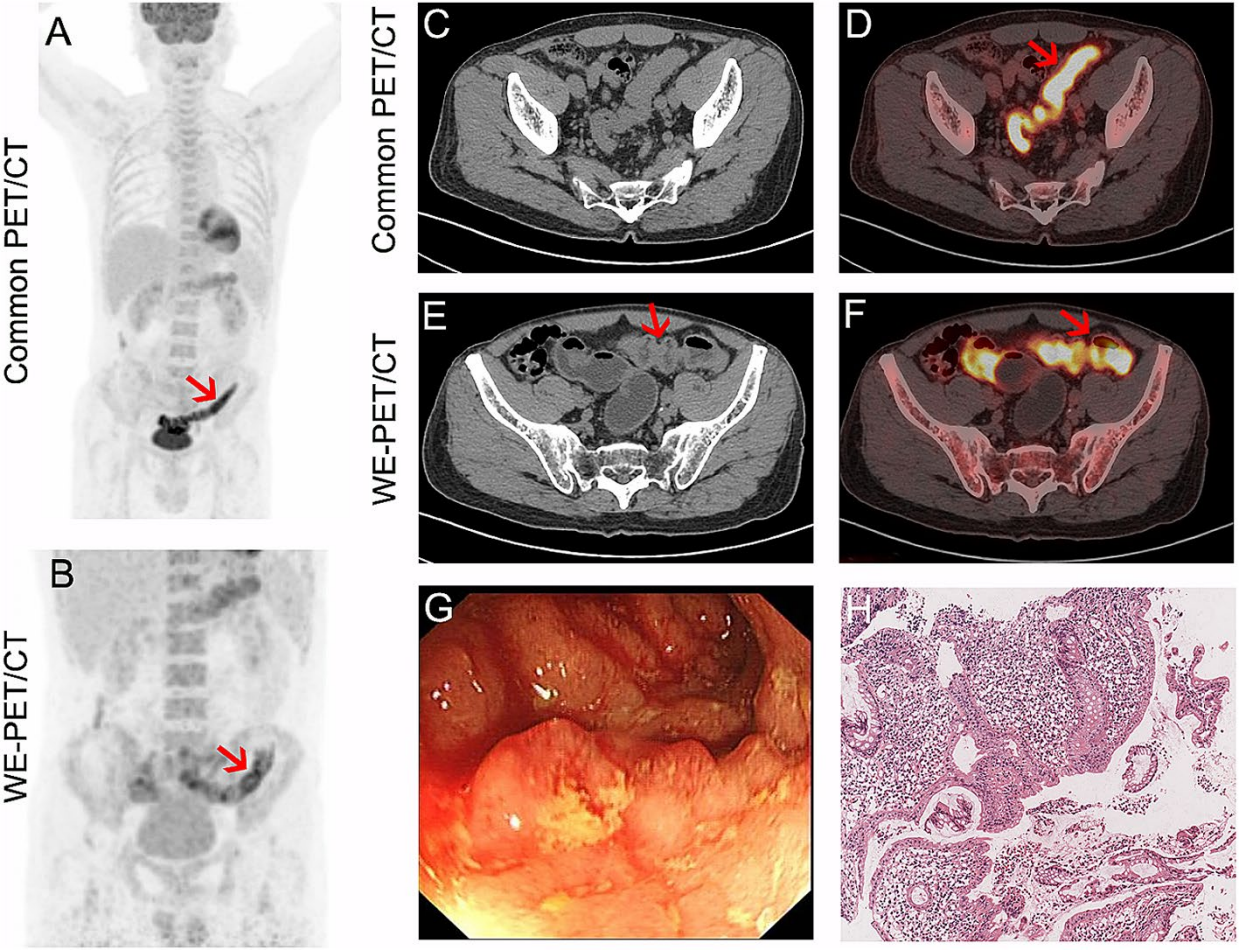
A 61-year-old male patient with IgG4-related pancreatitis. Segmental ^18^F-FDG uptake in the sigmoid was observed on common PET/CT (A, C and D). The ^18^F-FDG uptake in the sigmoid was reduced on WE-PET/CT, with mild and uniform wall thickening (B, E and F, red arrow). The colonoscopy (G) and pathological analysis (H) confirm ulcerative colitis
